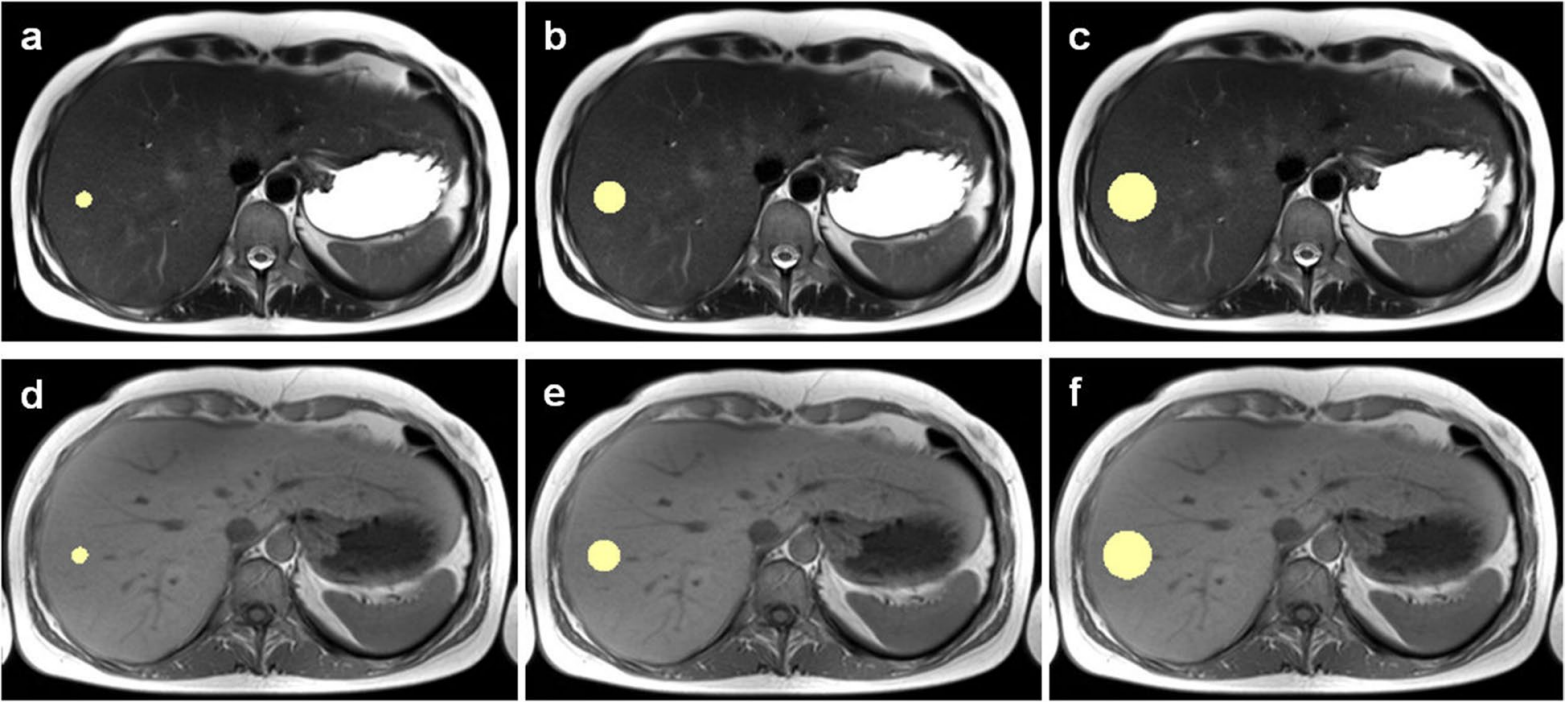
Volume of interest placement in the original images. Sphere-shaped volumes of interest of 10 mm (a, d), 20 mm (b, e), and 30 mm (c, f) diameter were placed in the right liver lobe attempting to exclude adjacent vessels. a–c T2-weighted turbo spin-echo sequences and (d–f) T1-weighted gradient-echo sequences, both of the same patient acquired on a 3-T scanner (scanner #2)
